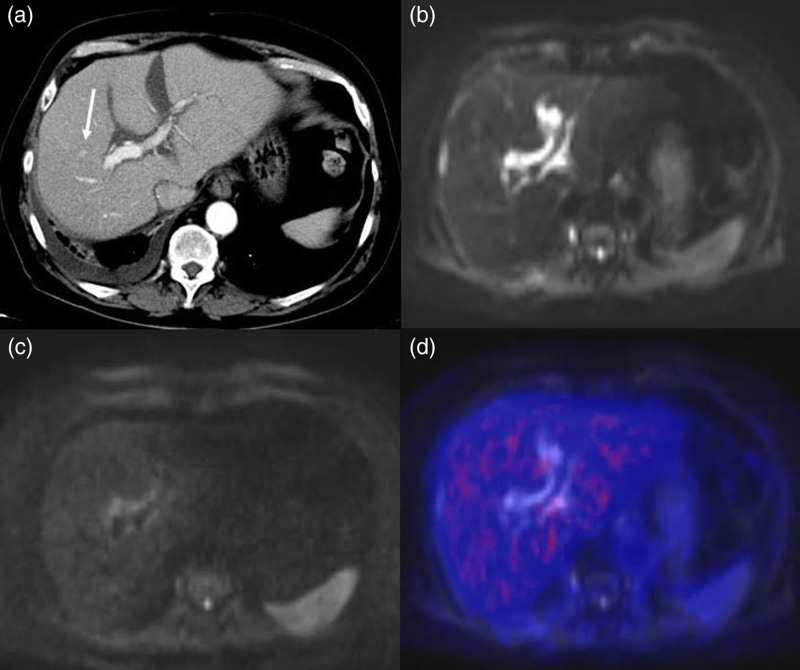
Contrast-enhanced CT scan showing a hypervascular nodule (arrow) in the S8 segment of the liver (a), suspect for hepatocellular carcinoma (HCC) recurrence; the lesion showed no restriction in the DWI sequences (b,c) nor hypermetabolism in the PET/MR fused images (d). At follow-up, it was confirmed to be a benign lesion.
